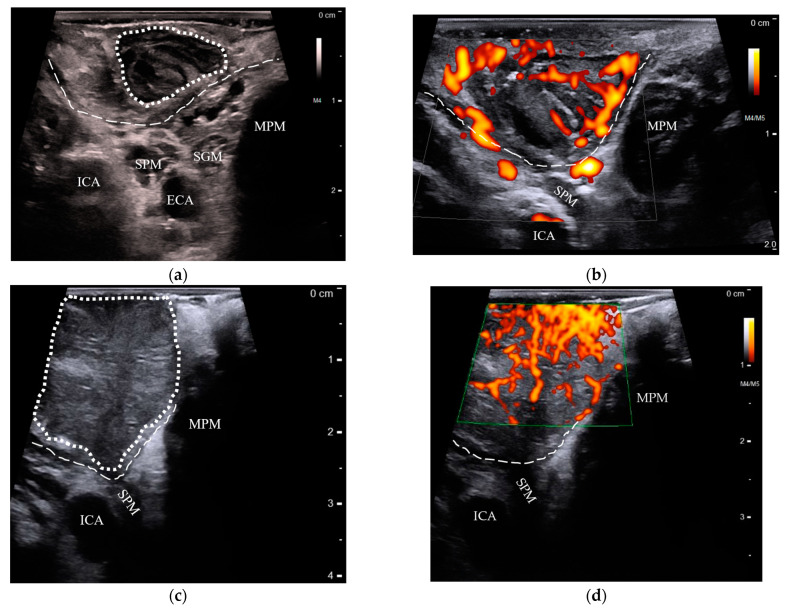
Comparison of transoral US features in benign and cancerous palatine tonsils: (a) a normal palatine tonsil (dotted outline) with striated crypts overlying the constrictor muscle (dashed line), lying superficially to the stylopharyngeus muscle (SPM), styloglossus muscle (SGM), medial pterygoid muscle (MPM), internal carotid artery (ICA), and external carotid artery (ECA); (b) power doppler shows the palatine tonsils’ blood flow originating from the constrictor muscle and branching outward parallel to the crypts; (c) a stage-T2 palatine tonsil HPV+ SCC appears as an enlarged, hypoechoic mass without crypt striations (dotted outline), but respects the boundaries of the constrictor muscle (dashed line); (d) with power doppler, the same tumor is seen with random, chaotically increased blood flow. US: ultrasound; HPV+ SCC: human papillomavirus-positive squamous cell carcinoma.
